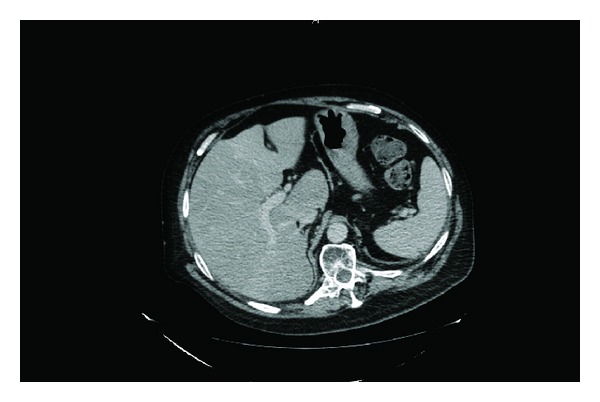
Axial section of computed tomography of abdomen revealed mild hepatomegaly and fatty changes in liver with focal sparing.
